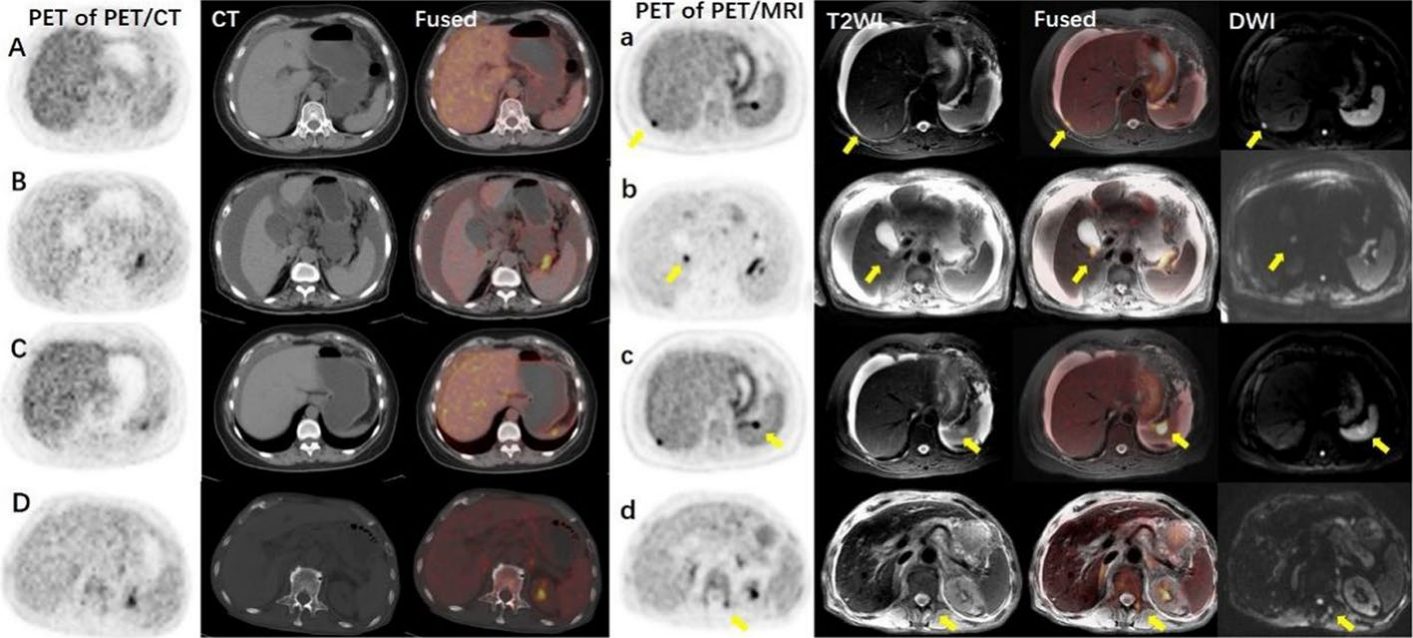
Small metastasis lesions (yellow arrows) detected by PET/MRI but missed by PET/CT. Line 1 (A and a) A 60-y-old woman with ovarian cancer. Peritoneal metastasis. Line 2 (B and b) A 75-y-old woman with ovarian cancer. Liver metastasis. Line 3 (C and c) Same case of Line 1. Spleen metastasis. Line 4 (D and d): A 65-y-old man with pancreatic cancer. Lumbar metastasis
